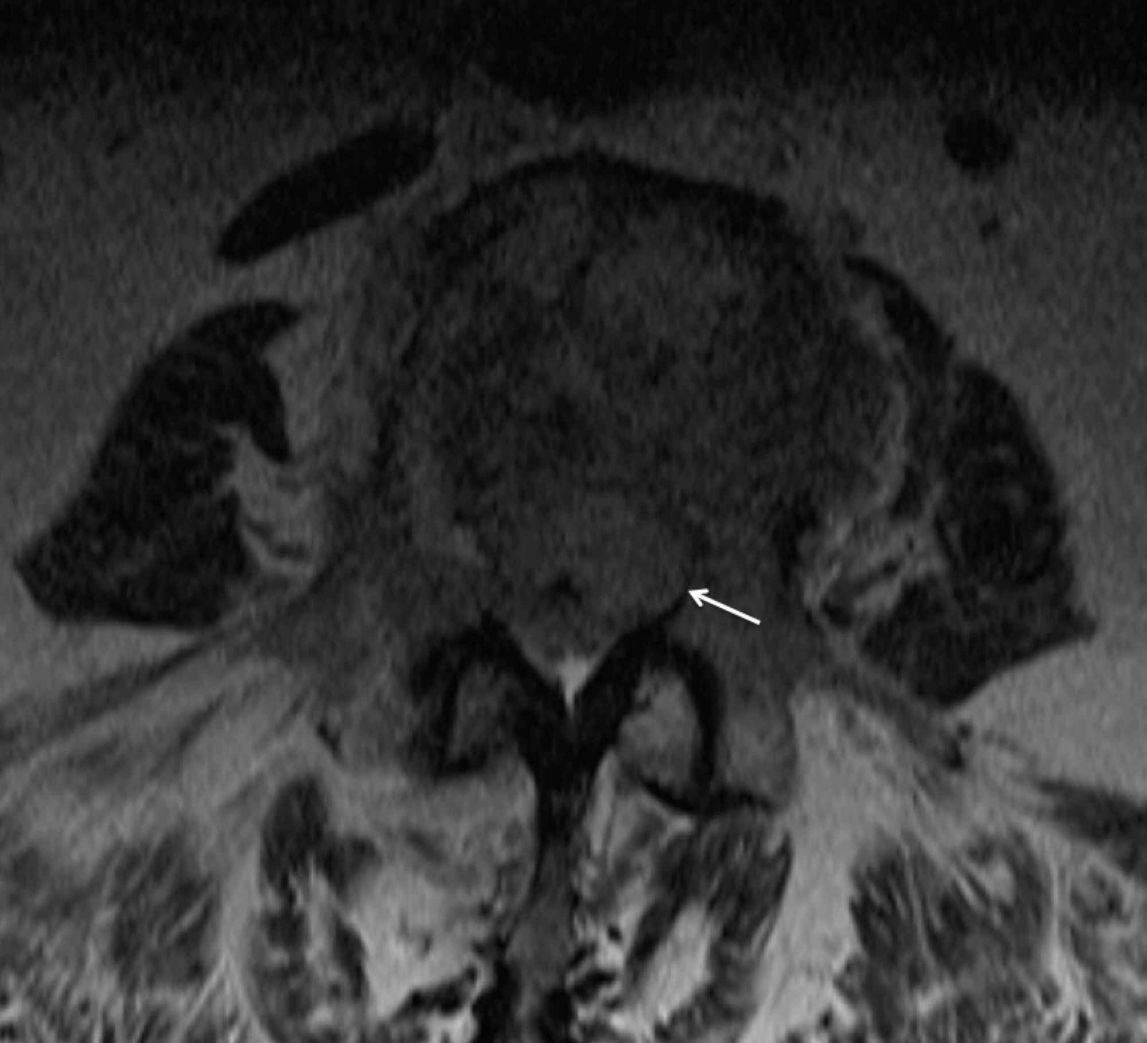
Axial T2-weighted image demonstrating a soft tissue mass extending into the epidural space (white arrow) that results in moderate central canal stenosis.
